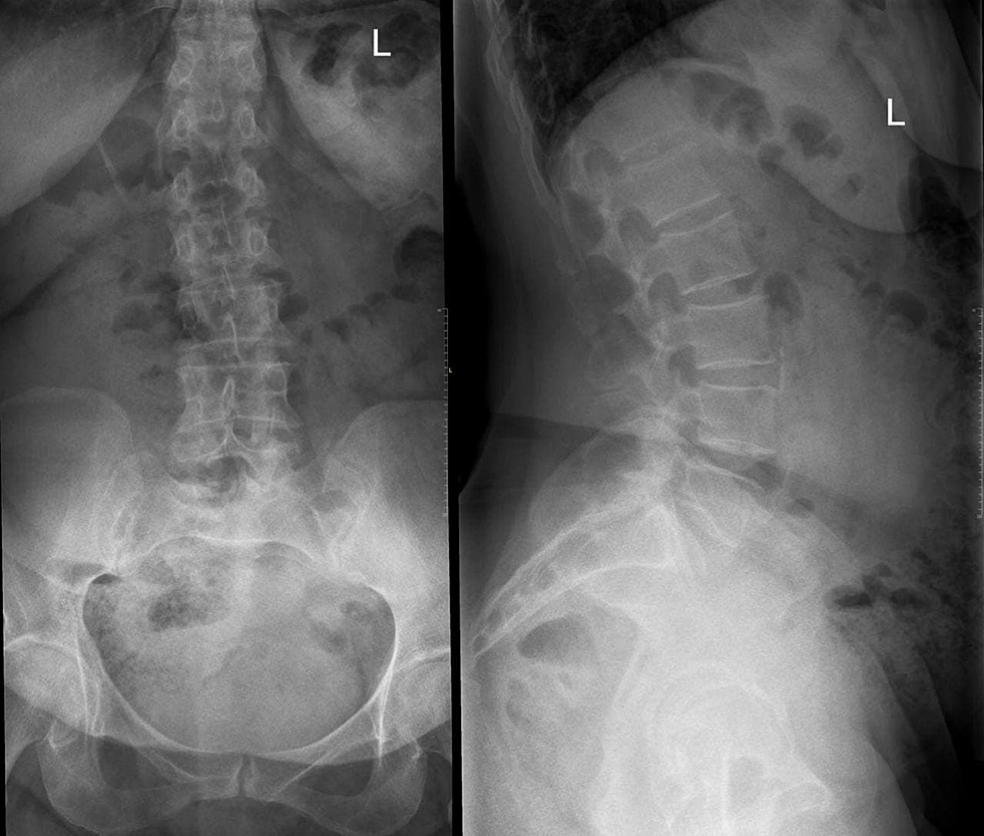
X-ray of the lumbar spine; anterior-posterior (AP) and lateral views Showing unremarkable lumbar spine
